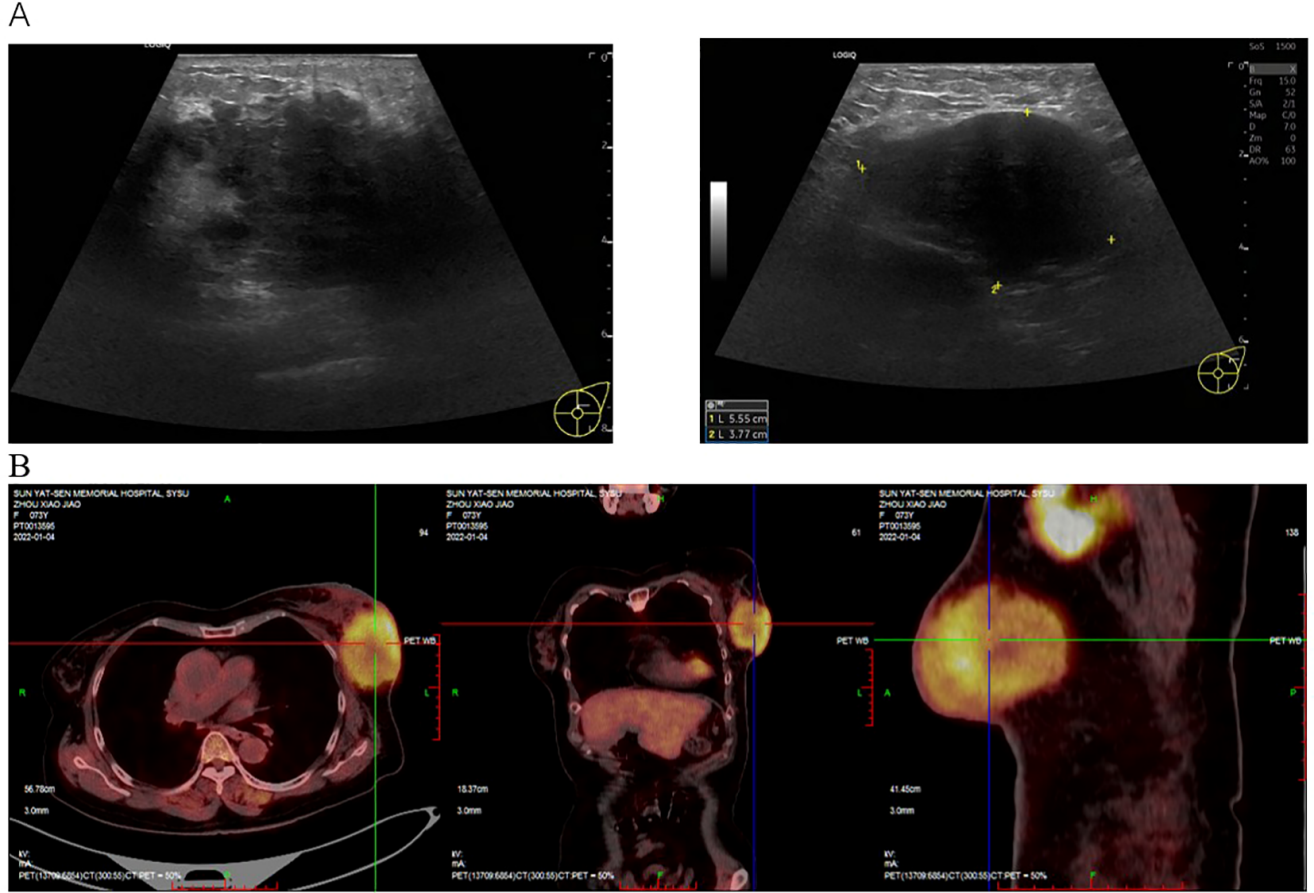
Imaging findings at the patient’s initial visit. (A) Breast ultrasound reveals thickened skin over the left breast, subcutaneous tissue edema, and a large mass (approximately 5+ × 3.9 × 5+ cm in size). Multiple lymph node echogenic foci, the largest measuring 5.6 × 3.8 cm, suggestive of metastasis. (B) PET-CT reveals a mass in the outer quadrant of the left breast, suspected to be breast cancer, with multiple lymph node metastases.
